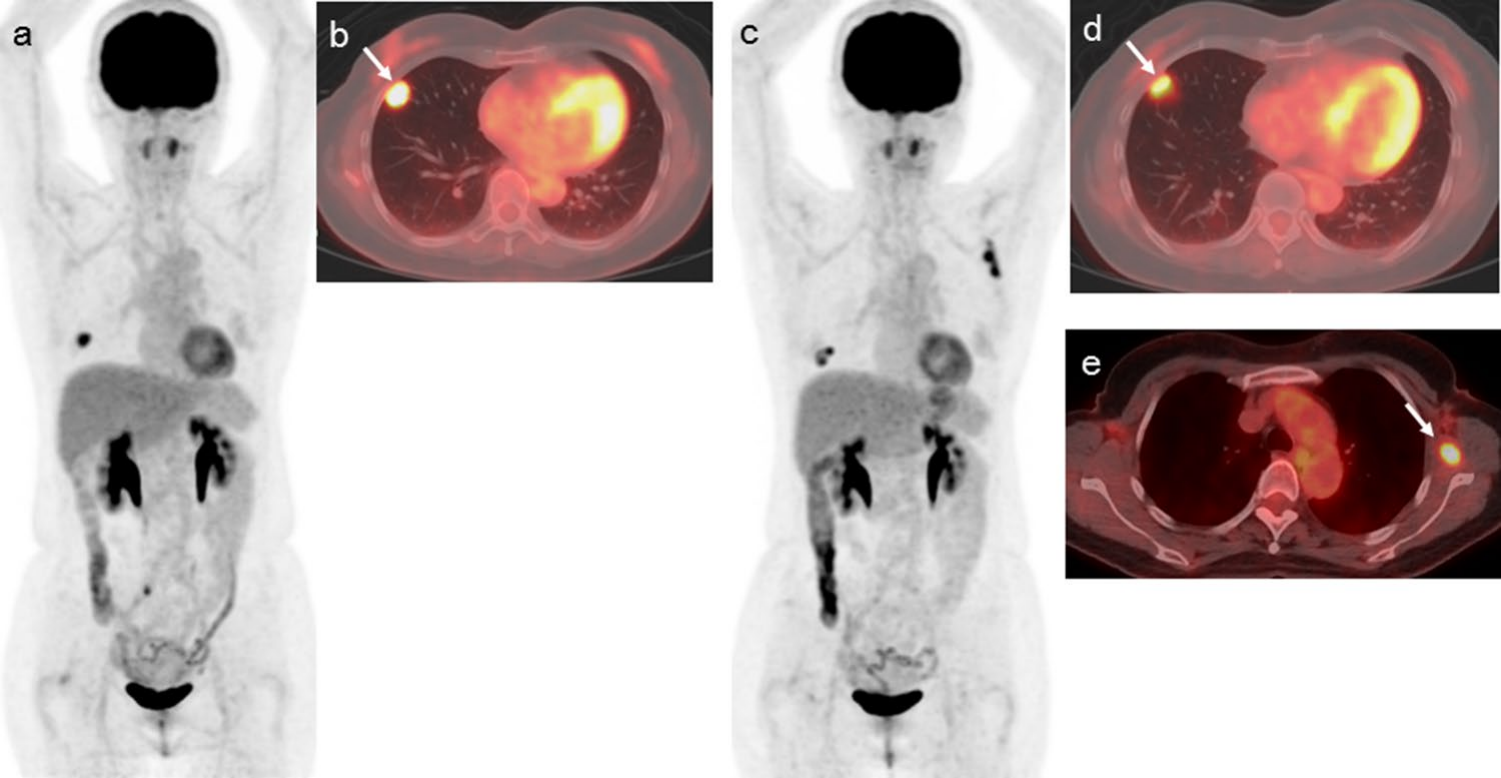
A 58-year-old woman with post-operative and chemotherapeutic recurrence malignant melanoma with lung metastasis received nivolumab. Baseline ^18^F-FDG PET/CT [MIP (a) and fused transaxial (b) images] shows abnormal ^18^F-FDG uptake in the right lung metastasis (b: arrow). The follow-up ^18^F-FDG PET/CT after 13 courses of nivolumab therapy [MIP (c) and fused transaxial (d, e) images] show the slight remission of known lung metastasis (d: arrow) with new appearance of subscapular muscle metastasis (e: arrow). The status was PMD according to harmonized EORTC criteria and harmonized PERCIST because of new lesions. Because the increase of the sum of the harmonized SUL_peak_ was 44.7% (from 3.87 to 5.6), the status was PMD according to harmonized imPERCIST. The patient exhibited progressive disease at 8.9 months and died 21.9 months after the initiation of nivolumab. Pretreatment harmonized SUV_max_, SUV_mean_, and SUL_peak_ of the right lung metastasis were 6.85, 4.50, and 3.87, respectively. Pretreatment harmonized whole-body MTV and TLG were 2.22 and 10.01, respectively. Posttreatment harmonized SUV_max_/SUV_mean_/SUL_peak_ of the right lung metastasis and left subscapular muscle metastasis were 4.21/3.08/2.44, and 5.53/3.81/3.16, respectively. Posttreatment harmonized whole-body MTV and TLG were 5.6 and 19.16, respectively
